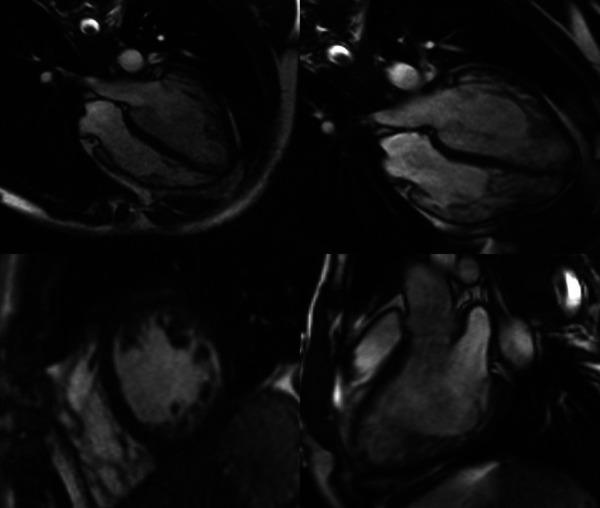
Cine cardiac magnetic resonance images of left ventricular cardiomyopathy with excessive trabeculation. Representative images of cine cardiac magnetic resonance imaging (CMR) steady-state free precession (SSFP) sequences at the time of diagnosis of cardiomyopathy with excessive trabeculation (left panel) and the onset of amiodarone-induced thyroiditis (right panel). The first row of images represents the four-chamber view through the horizontal long axis, whereas the second row consists of the short-axis pre-contrast scan (left panel) and vertical long-axis scan (right panel). Magnetic Resonance Imaging Scanner Siemens Magnetom® Avanto 1.5T (Siemens Medical Solutions, Inc., Hoffman Estates, IL, USA) was used to acquire CMR scans.
